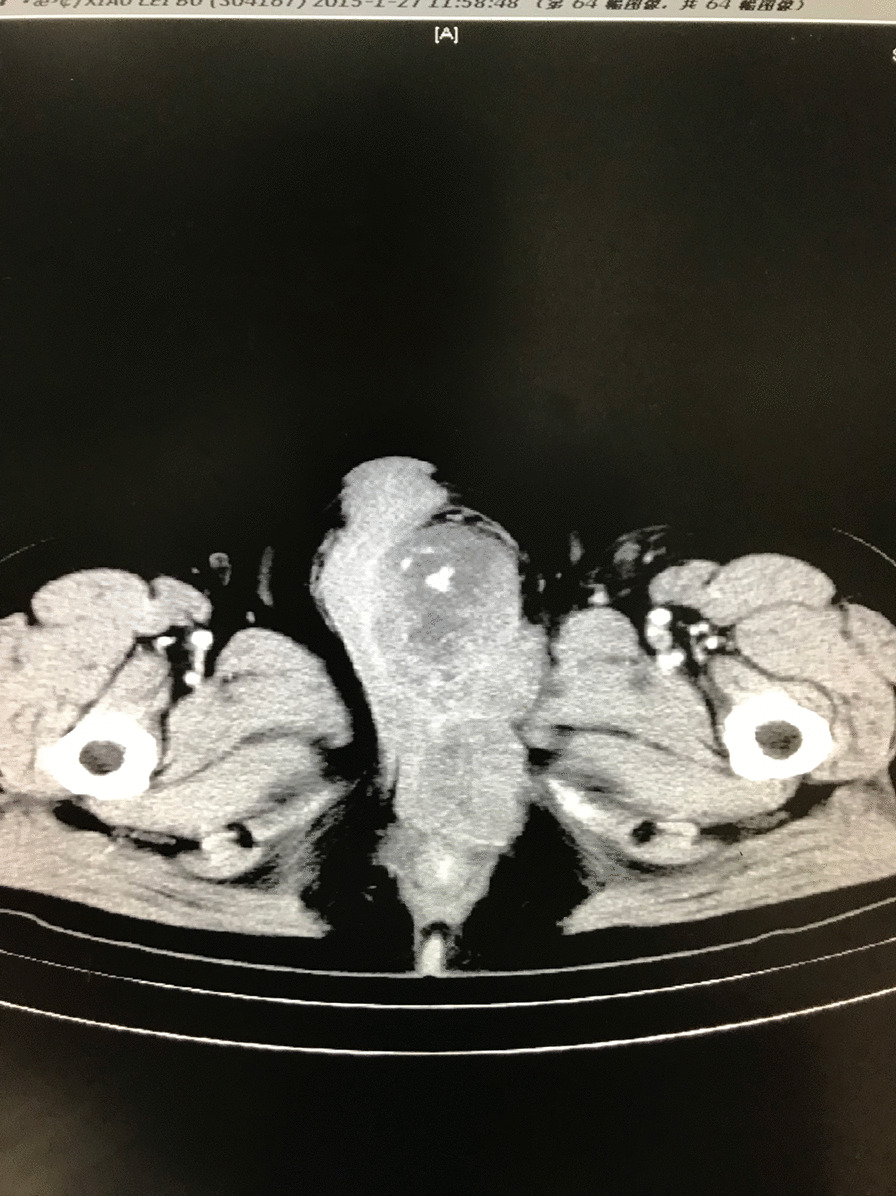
Computed tomography imaging. 5 months after surgery
